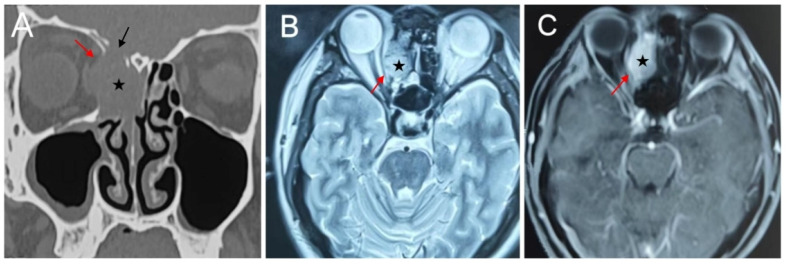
Imaging features of SMARCB1-deficient sinonasal carcinoma (Case 6). (A) Coronal noncontrast CT showing a tumor in the right ethmoid sinus (black star), extending through the lamina papyracea into the right orbit (red arrow) and eroding the ethmoidal roof (black arrow). (B) Axial T2-weighted MRI demonstrating mildly hyperintense signal in the right ethmoid sinus (black star). (C) Post-contrast T1-weighted MRI showing heterogeneous enhancement. The lesion compresses the medial rectus muscle (red arrow) and is closely related to the anterior skull base.
